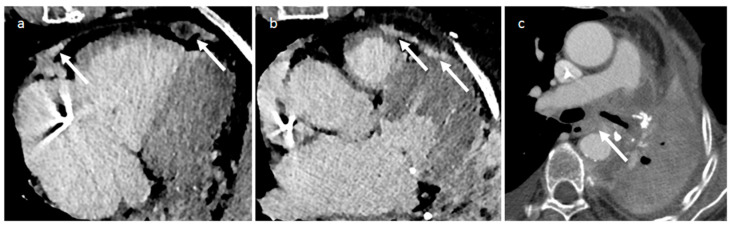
CT images of a 66-year-old female patient with multiple pericardial metastases (white arrows—images (a,b)) and lymphadenopathies (white arrow—image (c)) who previously underwent left lobectomy for lung adenocarcinoma.
